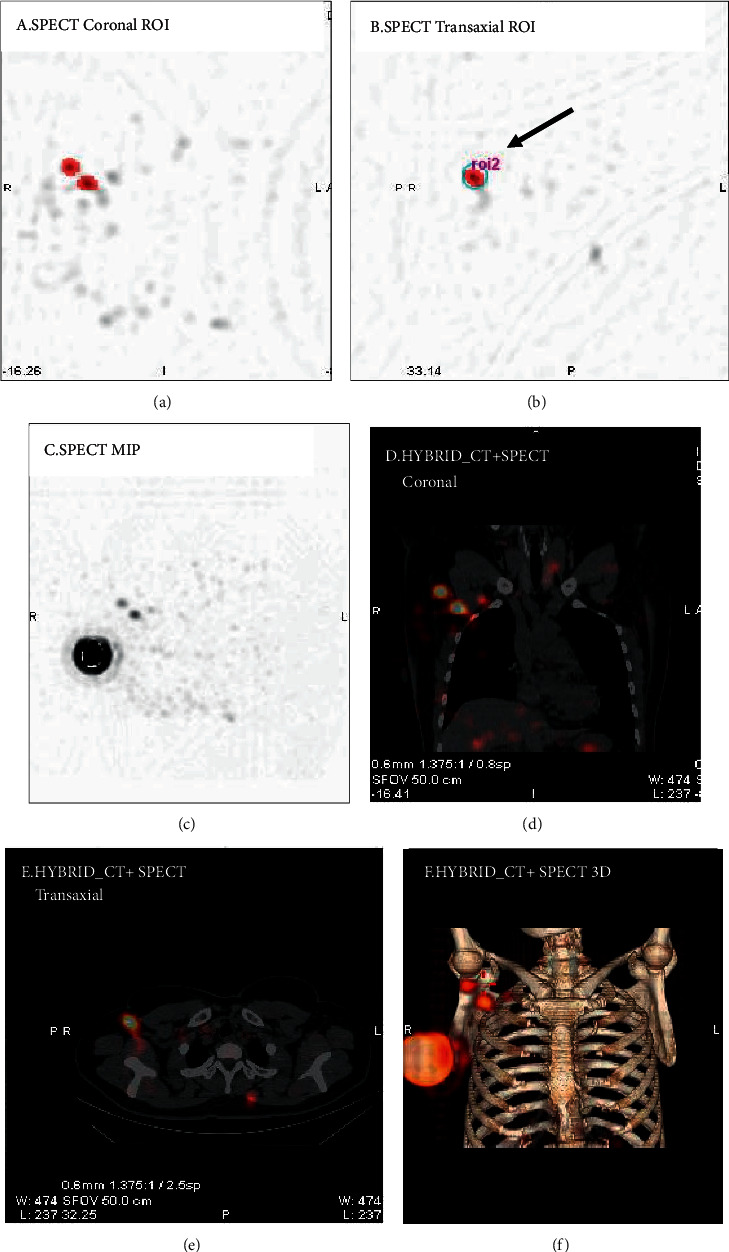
Graphical images describing the semiautomatic segmentation for the region of interest (ROI) and generation of radioactivity counts of hot nodes. (a–f) Coronal, transaxial, and MIP views of SPECT and corresponding hybrid SPECT/CT images.
